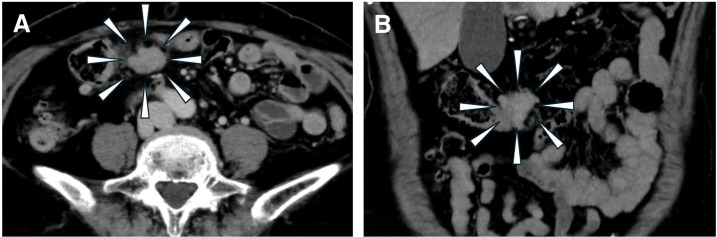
Contrast-enhanced CT of the abdomen. Axial (A) and coronal views (B). A 40-mm tumor borders the middle of the transverse colon, with a pale contrast effect.
